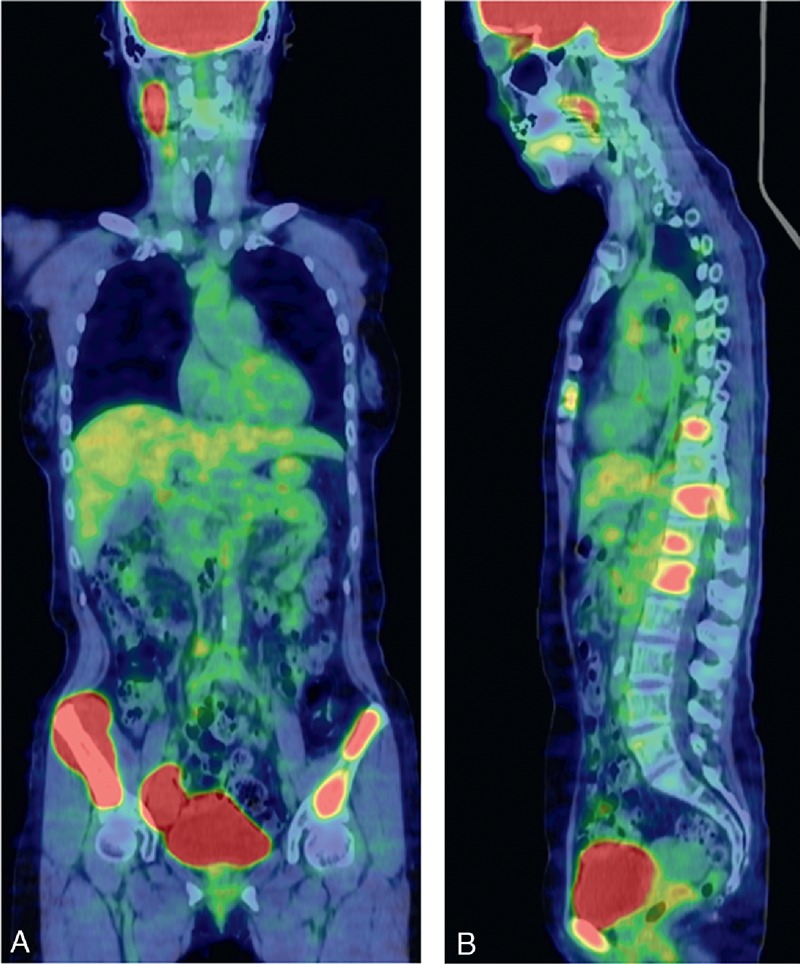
The result of ^18^F-FDG-PET scanning at transformation. ^18^F-FDG accumulated in the iliac bone, left pubic bone, thoracic vertebrae, right neck and flank, and right iliac lymph nodes. Red signals indicate accumulation of ^18^F-FDG, suggesting high tumor proliferation. The maximum standardized uptake value was 18.7 in the right iliac bone. (coronal view [A], sagittal view[B]). ^18^F-FDG-PET = fluorine-18 fluorodeoxyglucose positron emission tomography.
